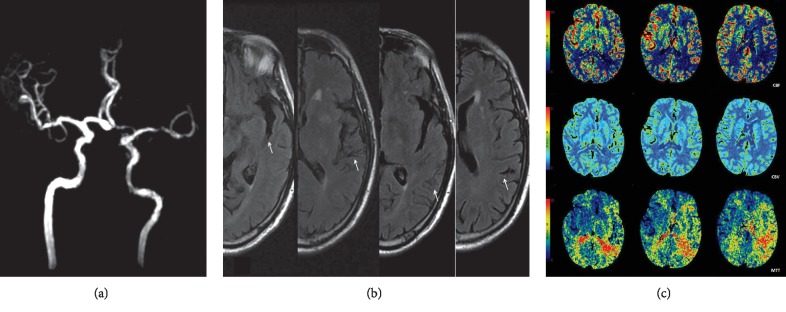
Fluid-attenuated inversion recovery vascular hyperintensity in a patient with left middle cerebral artery severe stenosis (M1). (a) MRA showed severe stenosis of the left MCA M1. (b) Dot-like and serpentine hyperintense signal of the middle cerebral artery branches in the left Sylvian fissure and the sulci of the left fronto-parietal lobes (arrows). (c) CT perfusion imaging showed severe hypoperfusion with prolonged MTT and decreased CBF and CBV values in bilateral cortical border zones and the left side of MCA territories.
